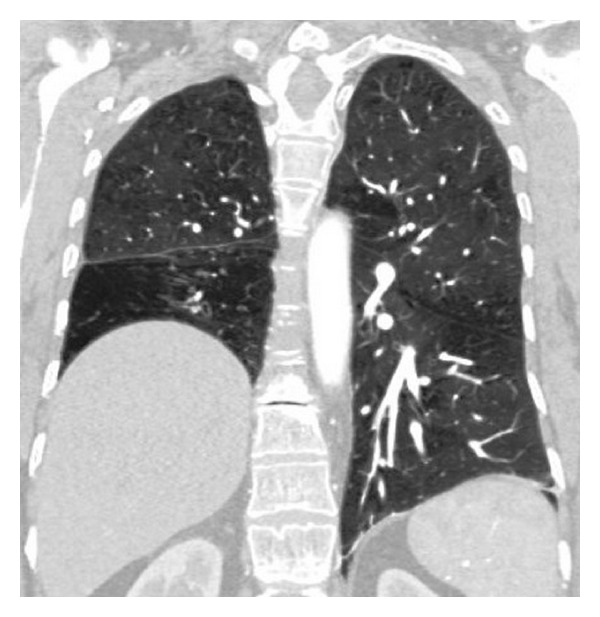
On coronal CT image, emphysema and parenchymal scars were seen in the lower lobe of the right lung.
